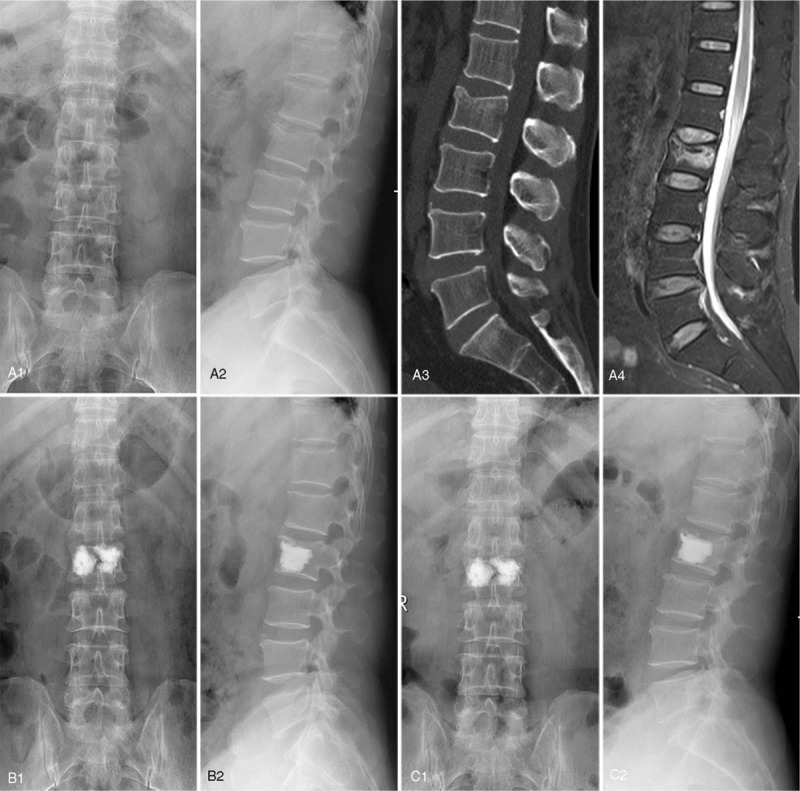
A 57-year-old male diagnosed as L2 OVCF with “H” shaped cement filling pattern. Preoperative X-ray from coronal plane (a1) and sagittal plane (a2), CT image (a3), and MRI fat suppression image (a4). Postoperative X-ray from coronal plane (b1) and sagittal plane (b2) at 2-days follow-up. Postoperative X-ray from coronal plane (c1) and sagittal plane (c2) at 1-year follow-up.
